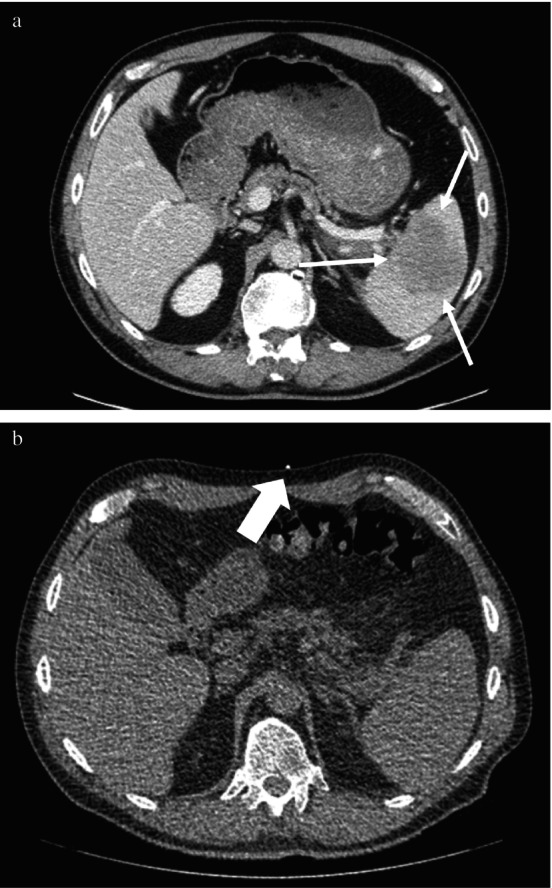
(a) Hypovascular, hypoattenuating splenic mass (white arrows) is seen within the splenic parenchyma. The spleen is not enlarged and is located posteriorly within the upper abdominal cavity. (b) Noncontrast CT imaging reveals that the splenic mass is isoattenuating with splenic parenchyma and cannot easily be visualized. Large white arrow indicates one of three MediView XR90 skin markers required for registration.
